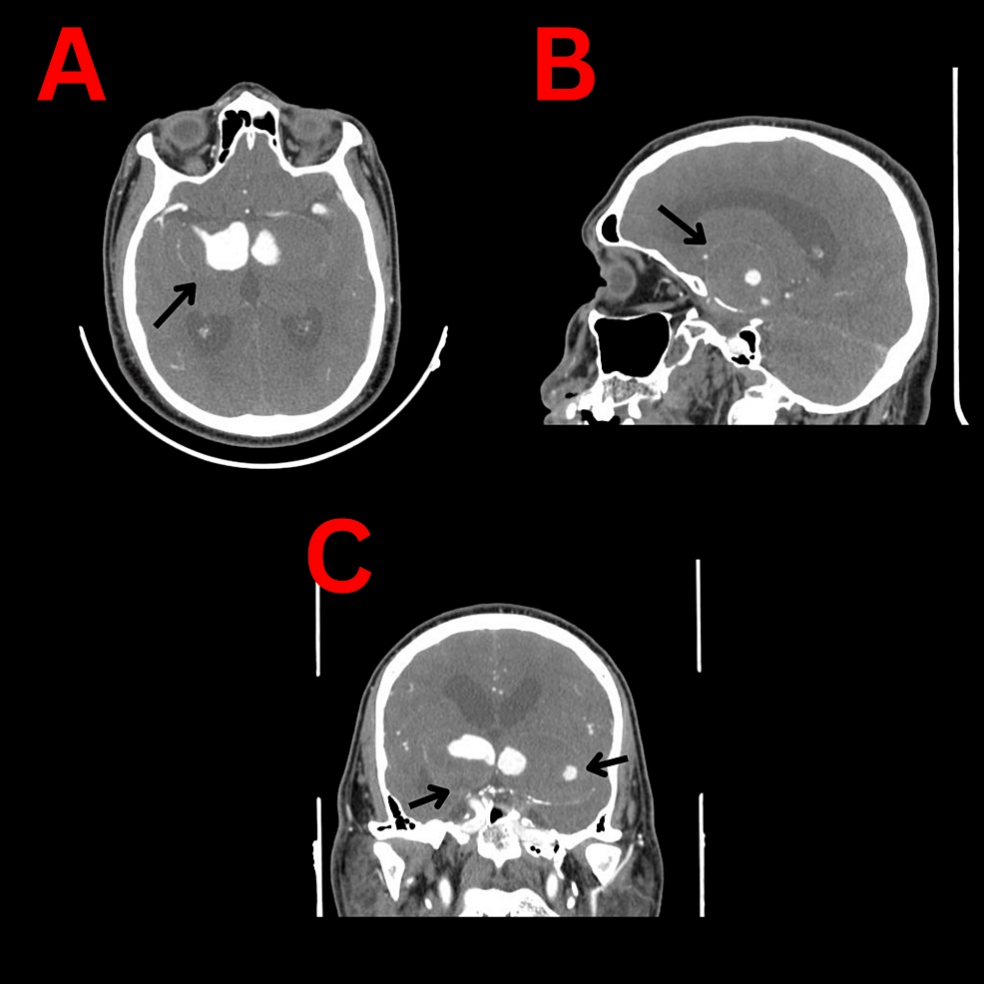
CT angiogram showing the bilateral huge aneurysm (the black arrows) A: Axial cut showing bilateral giant aneurysms of the internal carotid arteries . B: Sagittal view showing the aneurysm. C: Coronal CT angiogram cut demonstrating the bilateral partially thrombosed aneurysms.
